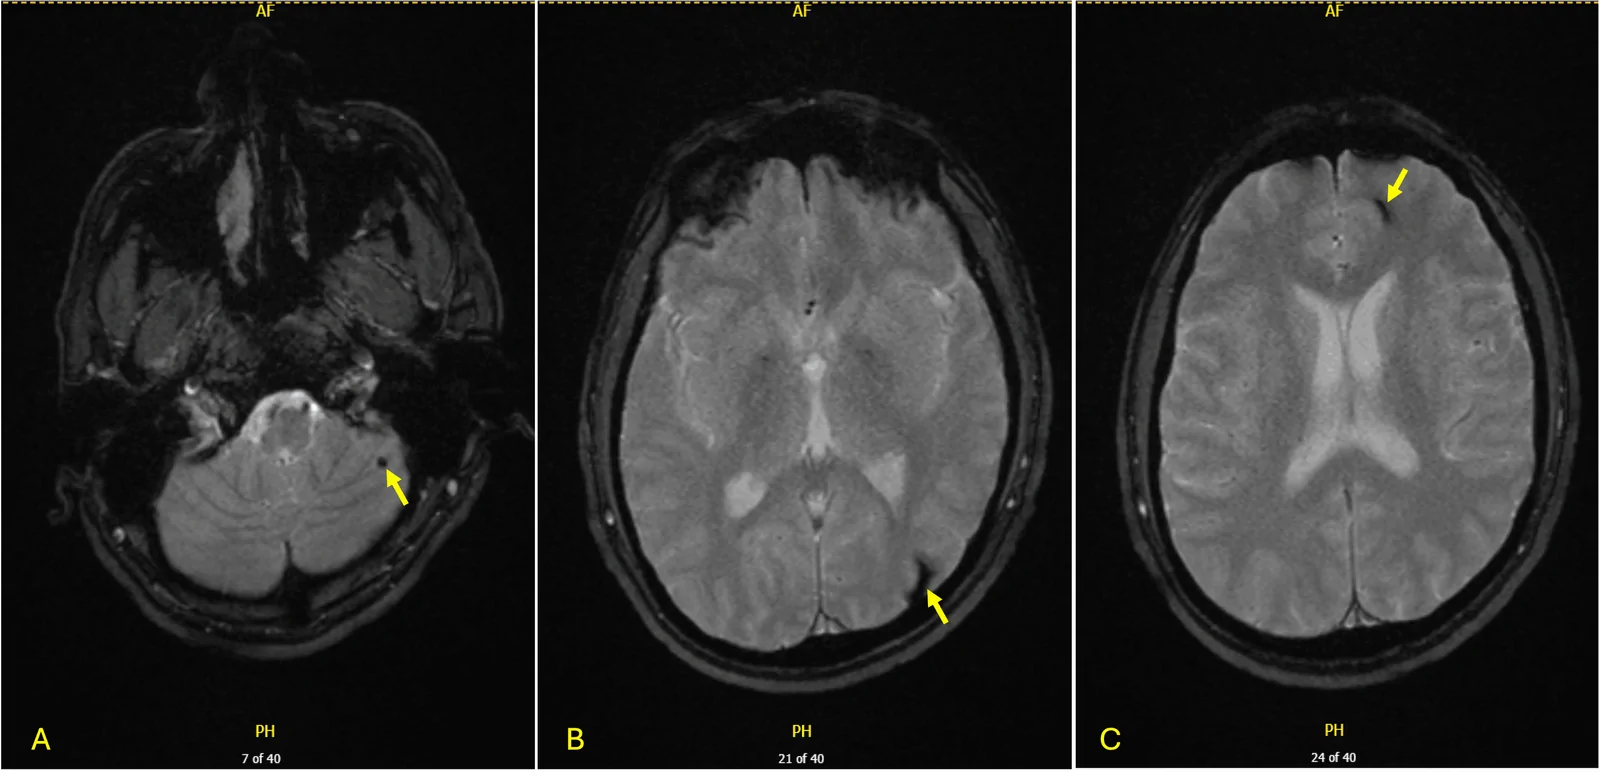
Day 2 MRI brain demonstrating focal susceptibility artifacts suggestive of chronic microhemorrhages Representative axial slices obtained during the first admission. Arrows indicate chronic microhemorrhages in the (A) left cerebellar, (B) left occipital, and (C) left frontal lobes. The multifocal, multilobar distribution of these chronic hemorrhagic lesions, together with the acute SAH in Figure 1, raised early suspicion for an underlying bleeding diathesis rather than a single focal vascular lesion.
